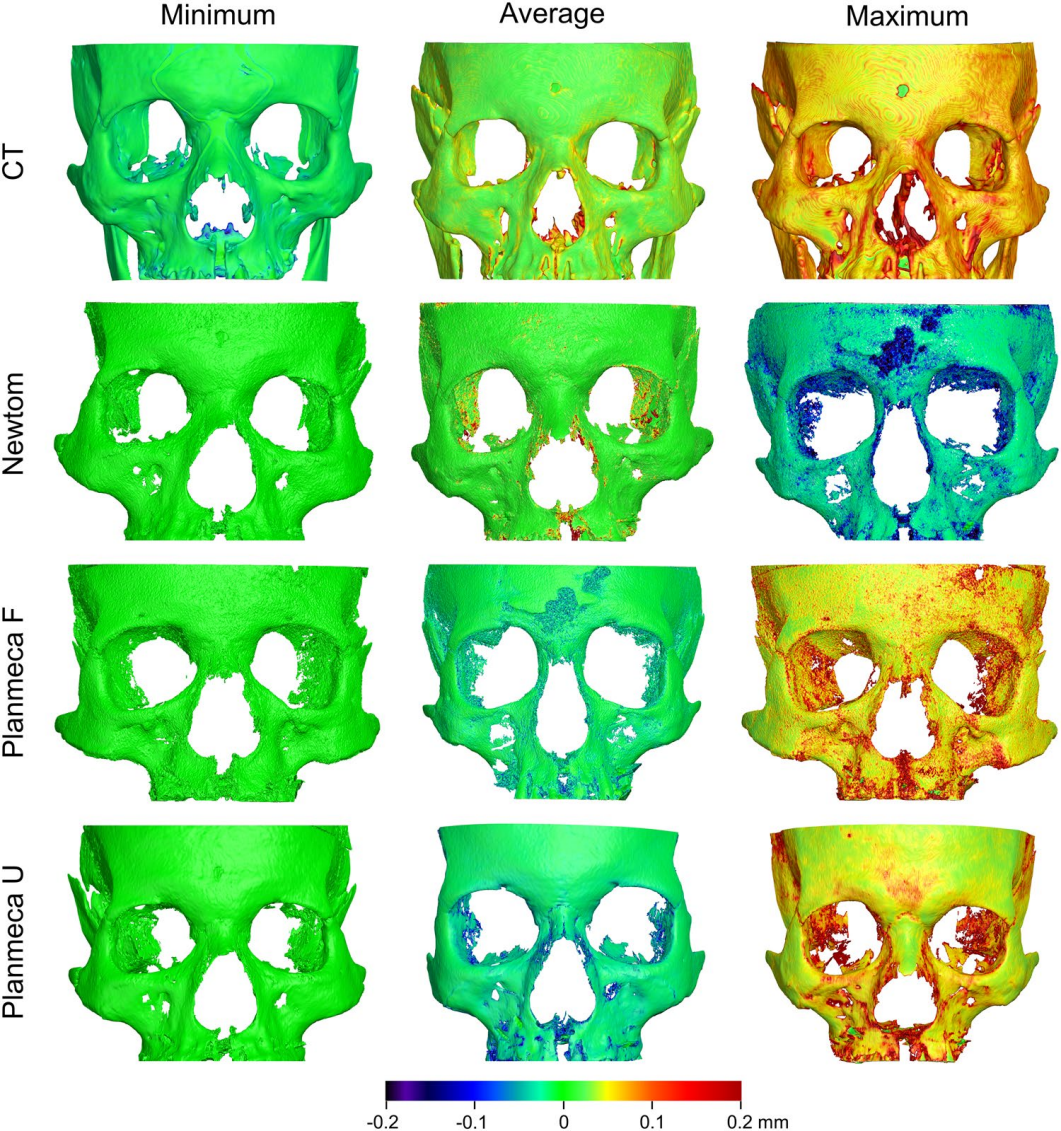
Colour coded distance maps between repeatedly segmented surface models by the same operator, representative of the minimum, average, and maximum differences detected in the sample for each acquisition setting. The compared models retained their original spatial relation within the source radiographic volume (status before superimposition).
